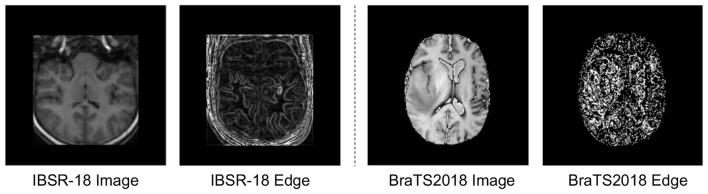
Representative MRI images and corresponding edge maps from IBSR-18 and BraTS2018. Compared with BraTS2018, IBSR-18 exhibits relatively clearer structural boundaries.
